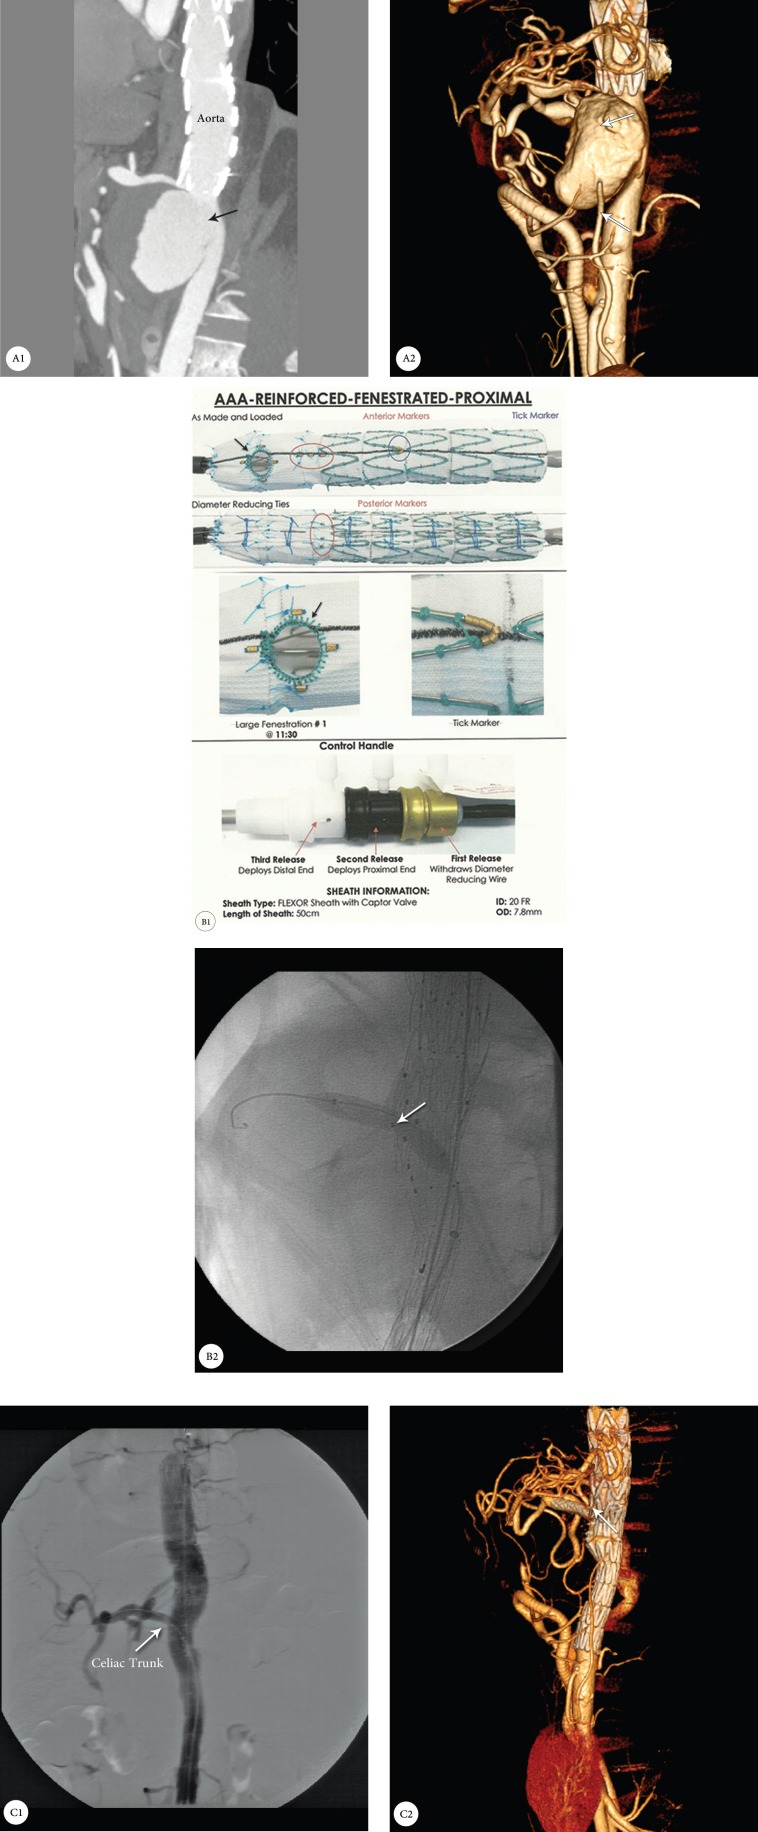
A1 & A2: Computed tomography angiography of the thoracoabdominal aorta, showing a pseudoaneurysm (arrows); B1: Location of the hole in the custom-made 1-hole stent graft (arrow); B2: Fluoroscopy in the lateral view, showing balloon inflation in the celiac trunk from the stent graft hole (arrows); C1 & C2: Postprocedural computed tomography angiography, showing fenestrated endovascular aortic aneurysm repair with the exclusion of the pseudoaneurysm and the perfusion of the celiac trunk (arrows).
